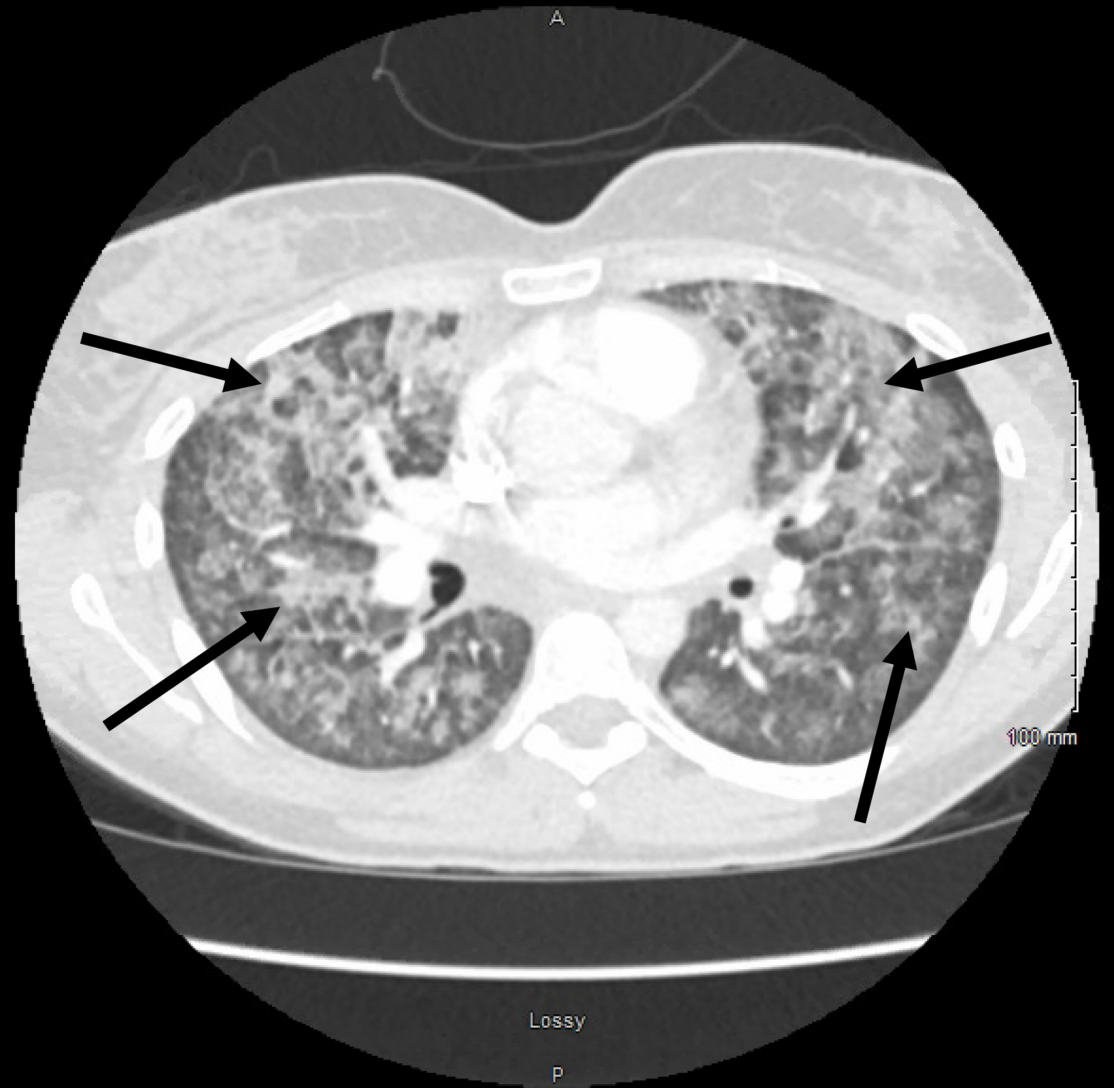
Chest CT (transverse plane image) showing diffuse bilateral pulmonary infiltrates (indicated by arrows).
